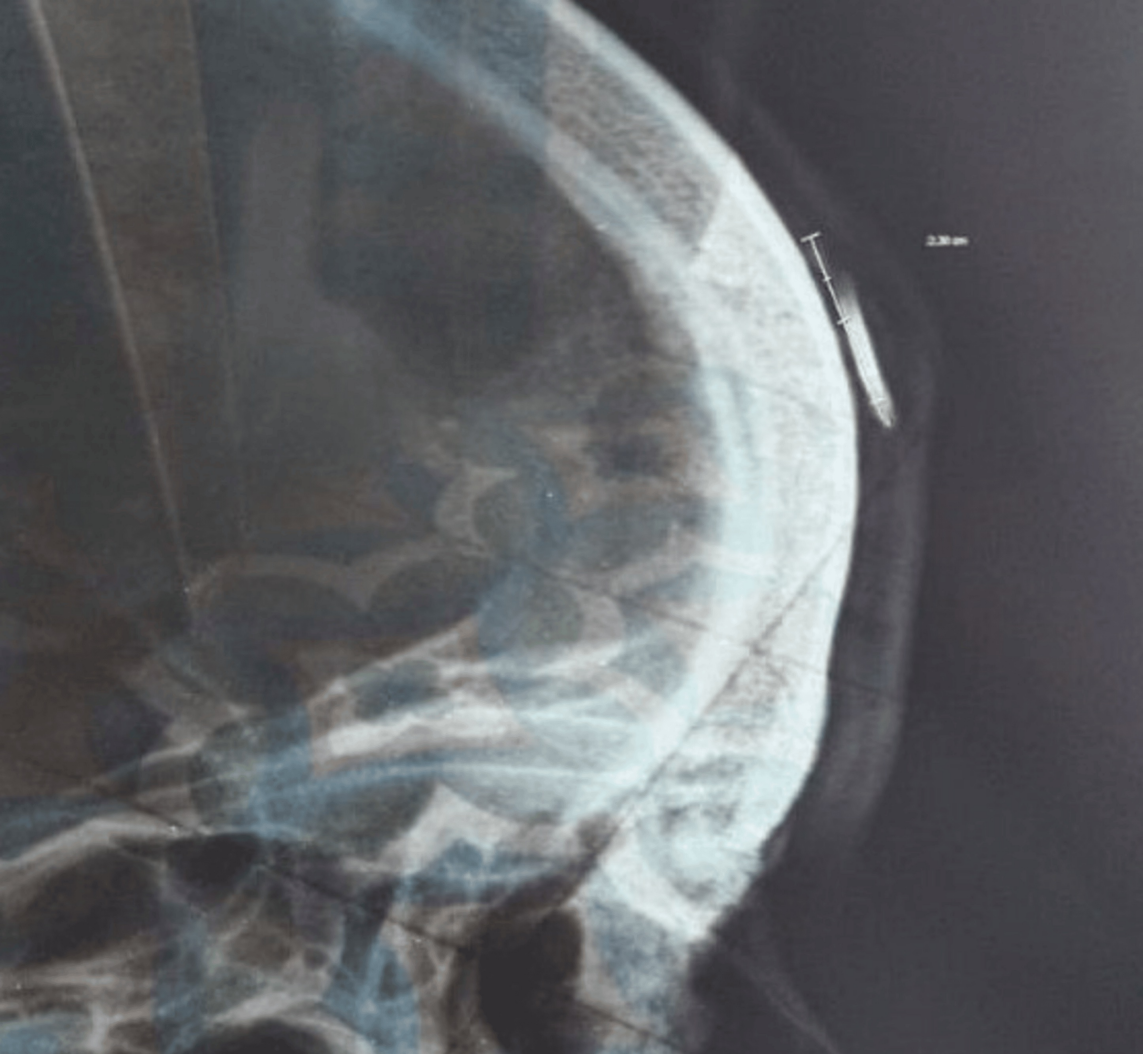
Lateral radiograph of the skull in the right frontal region (limited area radiograph)
